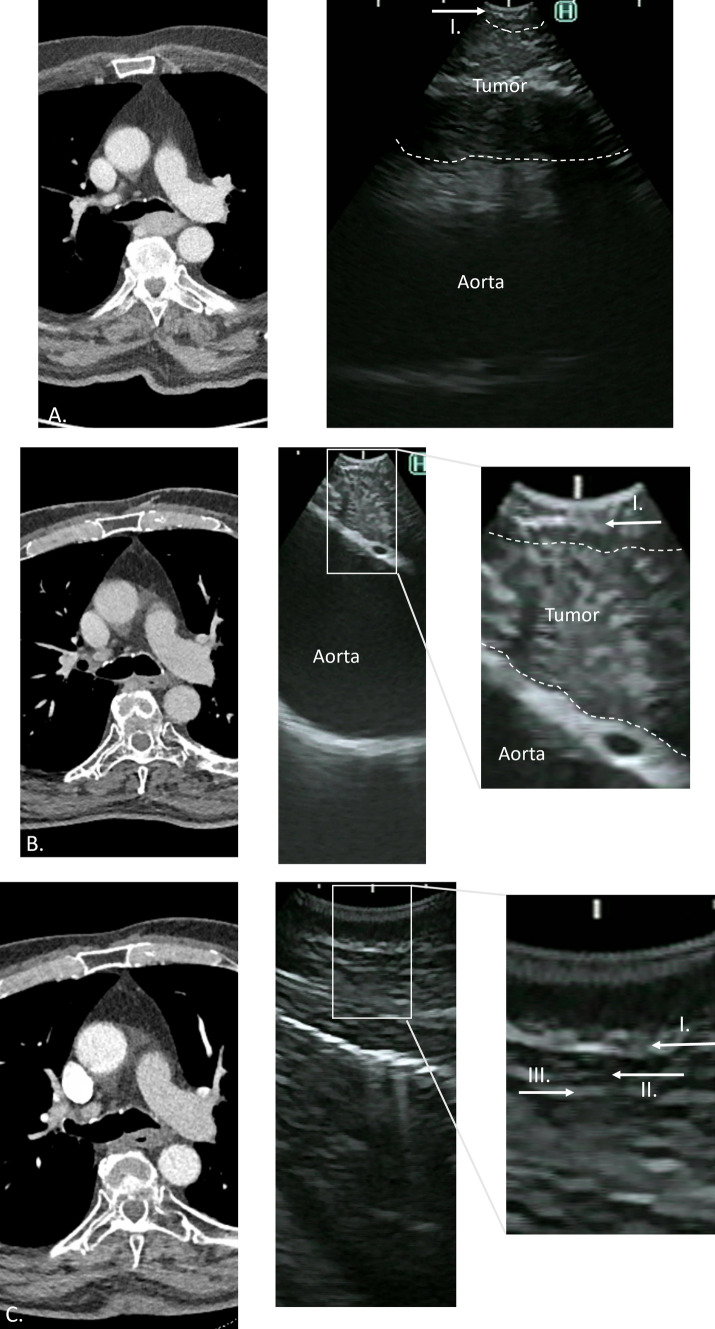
A A CT (left) image of a patient with suspicion of tumor invasion into the tracheabronchial tree before dCRT. On the EBUS (right) image, only the first of the three layers is recognizable on the EBUS, and tumor invasion in the tracheobronchial tree was considered to be present. B A CT (left) image of the same patient after dCRT. The EBUS (right) image shows an intact first layer, and tumor regression was observed. However, the adventitia (third layer) is absent, and persistent tumor invasion was considered to be present. C A CT (left) image of the same patient after an interval of 7 weeks. On the EBUS (right) image, the three layers are recognizable, and tumor invasion was considered to be absent. CT, computed tomography; dCRT, definitive chemoradiotherapy; EBUS, endobronchial ultrasonography
